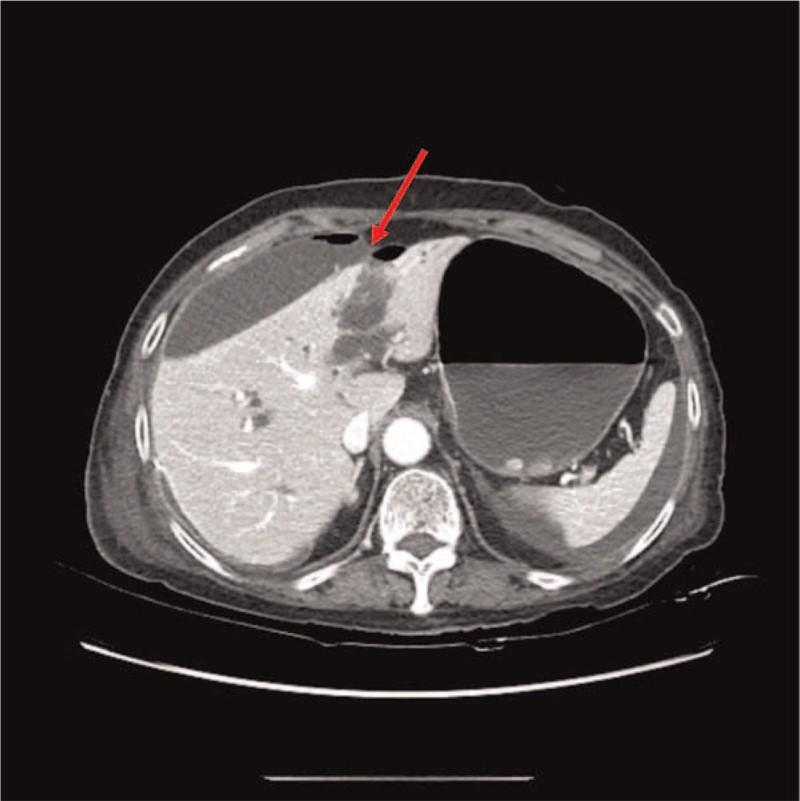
Postoperative computed tomography showing multiloculated fluid collection in perihepatic and perisplenic spaces. Red arrow indicates the point where bile leakage was found during surgery.
